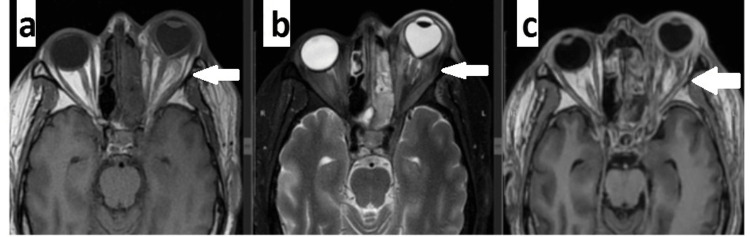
MRI T1W, T2W and T1W post-contrast images showing intraorbital extensions of the MM to the left eyeball (a) The MRI T1W image shows intraorbital extention and proptosis of left eyeball with distortion of the shape of the globe like the "shape of a guitar pick". (b) The MRI T2W image shows abnormal enhancement along the coats of the eyeball with posterior coning of the globe. (c) The MRI T1W post-contrast image shows extensive fat stranding at intra- and extraconal fat planes with muscle edema. All images are suggestive of signs of extensive orbital disease with an increase in intraorbital pressure. MM, mucormycosis
